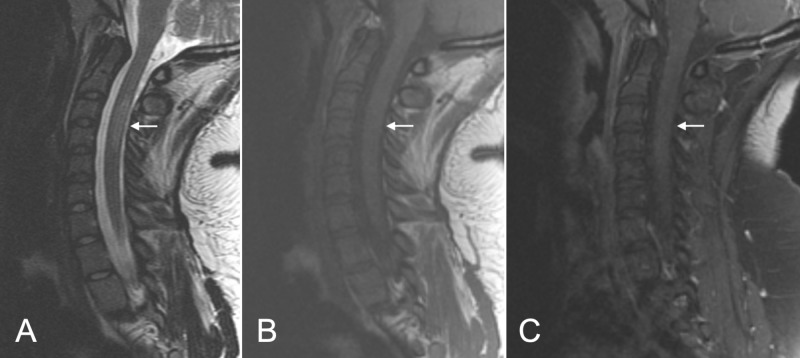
Follow-up MRI Follow-up MRI at two months shows mild reduction of the lesion size and intensity (arrow) on T2-weighted images (A) and T1-weighted images (B), with no signs of contrast enhancement (C).
